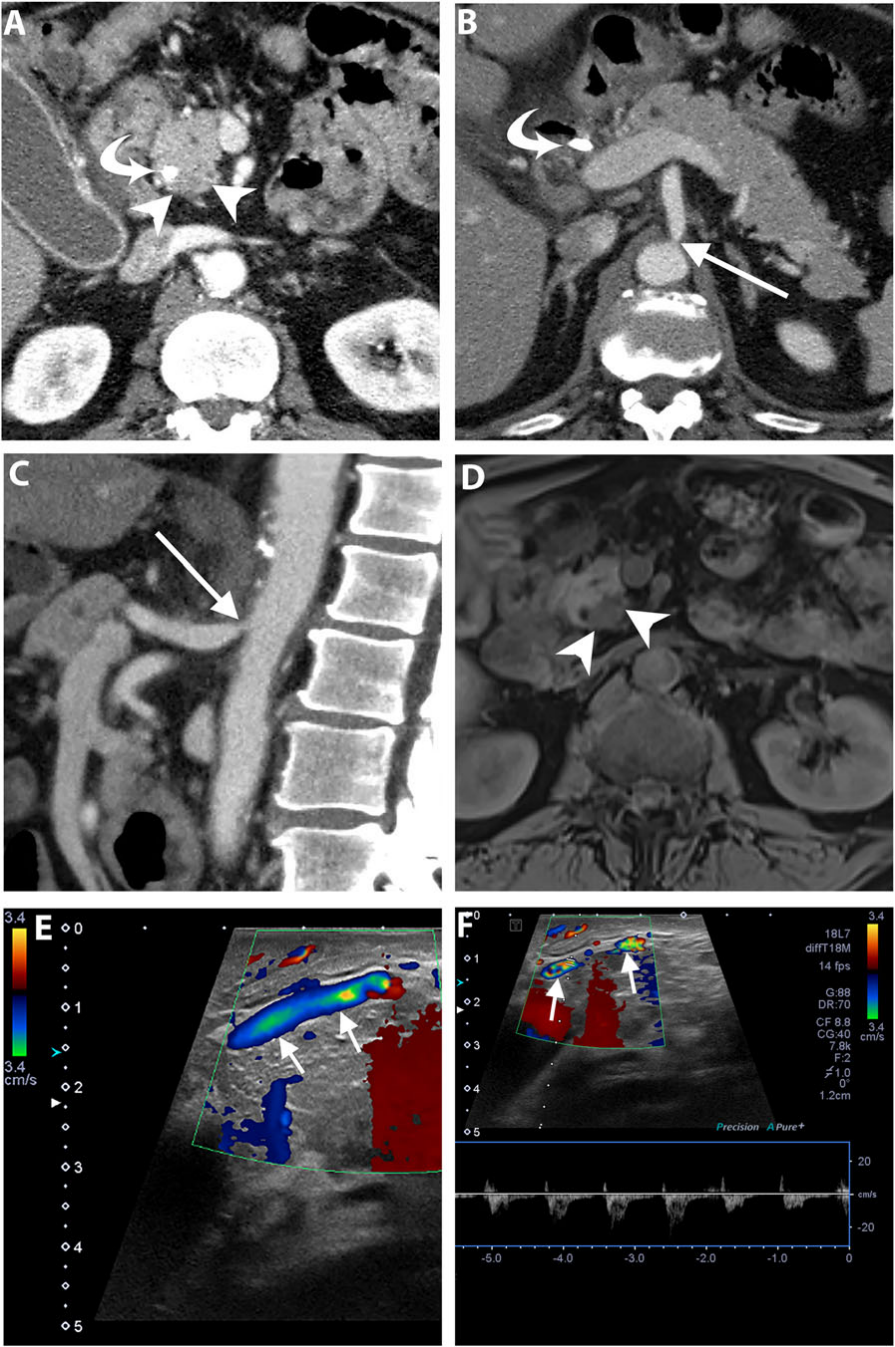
A 60-year-old man undergoing preoperative imaging for PDAC. Axial (a and b), and sagittal (c) pancreatic phase CT images show ostial stenosis of the celiac artery (arrow) due to atherosclerotic disease. The patient has a biliary stent (curved arrow) and PDAC is identified as a subtle hypoattenuating lesion (arrowheads). Axial fat-suppressed T1-weighted MR image (d) clearly demonstrates PDAC as a hypointense mass. Intraoperative Doppler ultrasound (e and f) shows a significant celiac artery stenosis by demonstrating caudocranial/reversed blood flow in the gastroduodenal artery, denoting its significant contribution to the hepatic arterial supply. The patient subsequently underwent pancreaticoduodenectomy after celiac artery stenting
